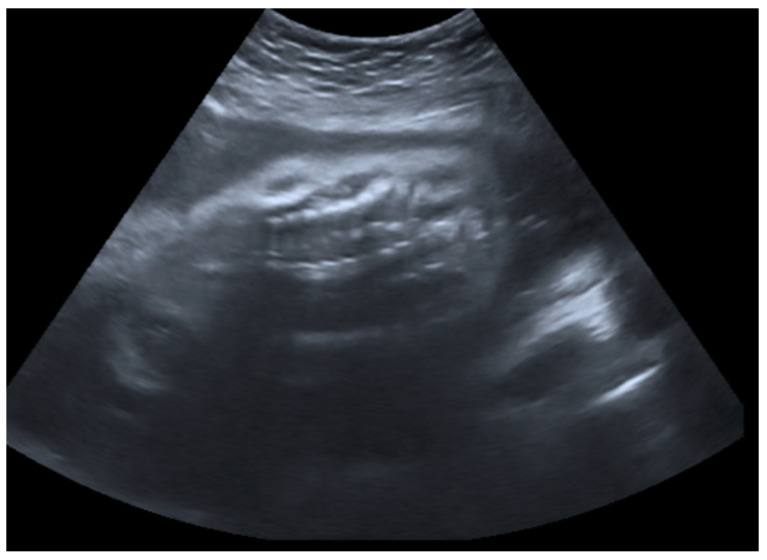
Transabdominal fetal ultrasound scan image including a coronal view of the fetal spine and chest. A deformed and compressed chest was observed.
